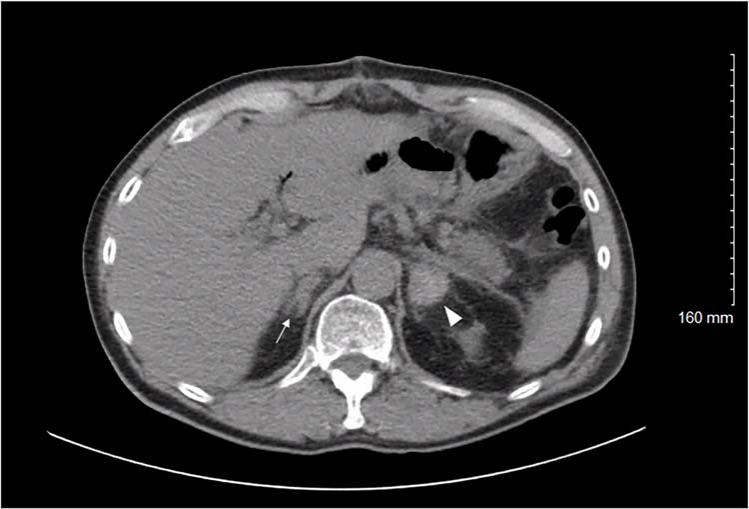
Axial CT abdomen/pelvis on POD 11 demonstrating the left adrenal gland (white arrowhead) with a high density 2.6 × 2.6 cm fluid collection; density is consistent with a subacute bleed. The right adrenal gland (white arrow) demonstrates thickening suggesting a small hemorrhage, less pronounced than the left.
